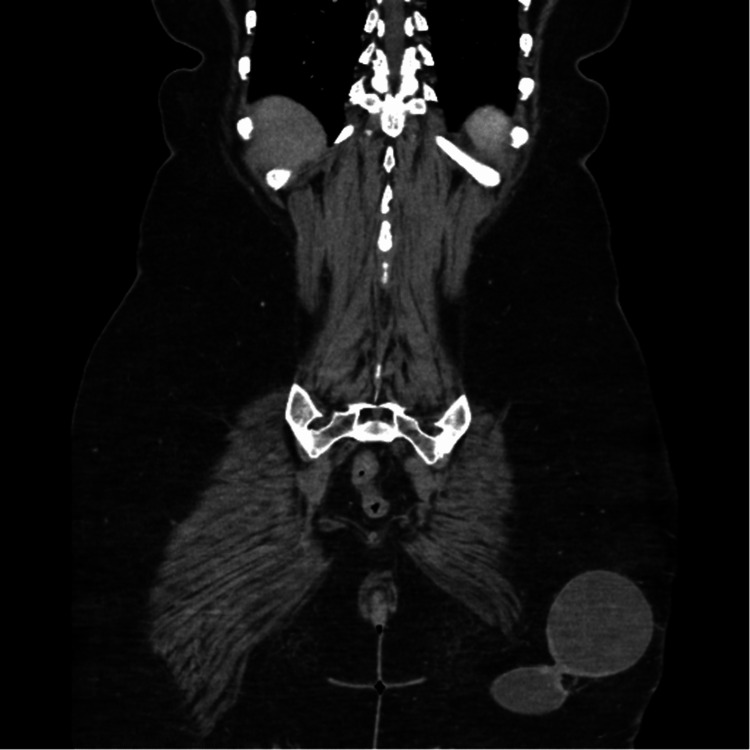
Sagittal CT scan showing a large, approximately 12.5 cm complex cystic trilobed mass in the subcutaneous left buttock soft tissues. The largest of the 3 lobes of this mass is located superiorly and laterally and measures 8.5 x 6.5 cm. A contiguous superior medial lobe measures 2.8 x 1.9 cm. A more inferior medial lobe measures 7 x 4.3 cm.
